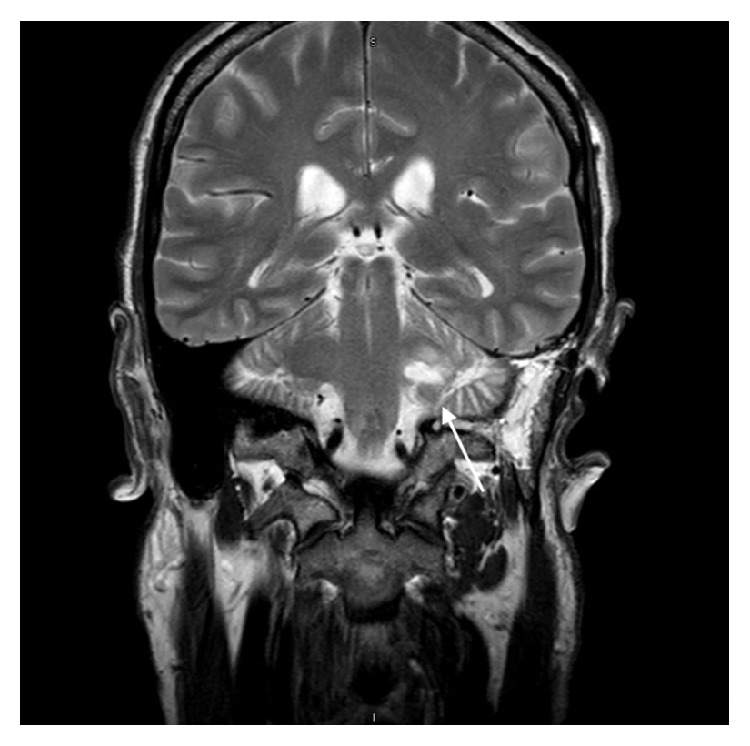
Coronal MR image (T2 weighted) showing the postoperative changes.
